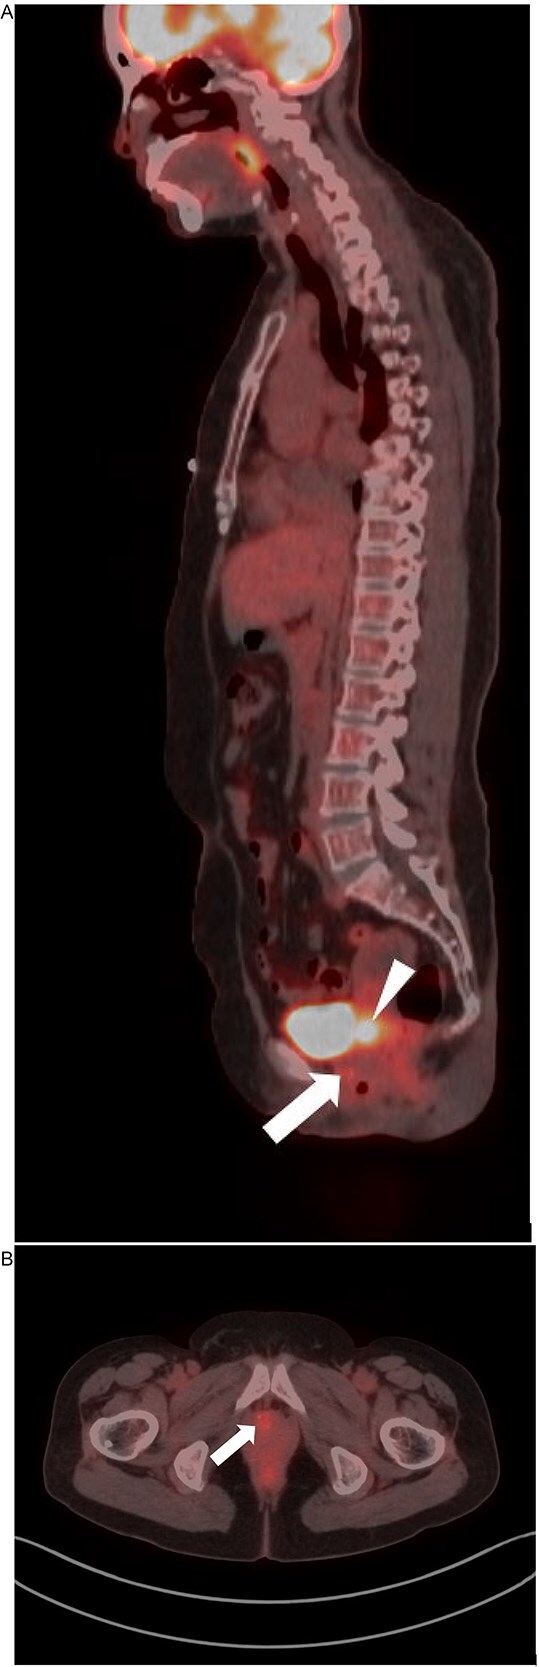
PET-CT: Sagittal (A) and axial (B) PET-CT demonstrates an approximately 2.2 cm uterine mass (arrowhead) with intense F-18 fluorodeoxyglucose (FDG) uptake, a finding that can suggest malignancy. There is an additional focus of FDG uptake in the cervix (arrow). There is normal physiologic FDG activity within the bladder.
